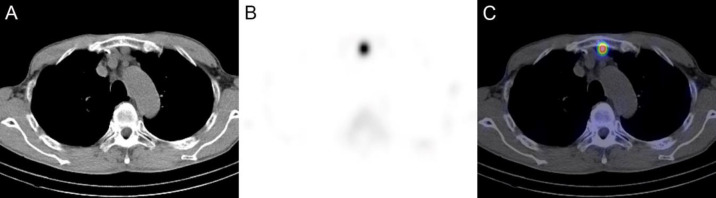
^99m^Tc-Sn-colloid SPECT/CT (A, plain CT; B, SPECT; C, fused SPECT/CT). Intense accumulation was observed in the anterior mediastinum, and the fused SPECT/CT images demonstrated that the accumulation corresponded to the nodule of interest
